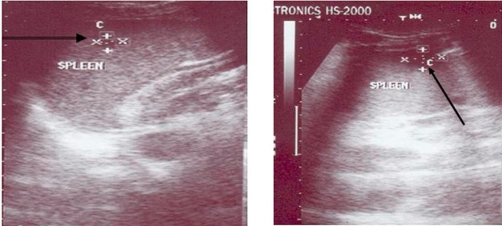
A pre-operative ultrasonographic study showing well-defined splenic focal lesions, cystic in nature and multiple in number.
